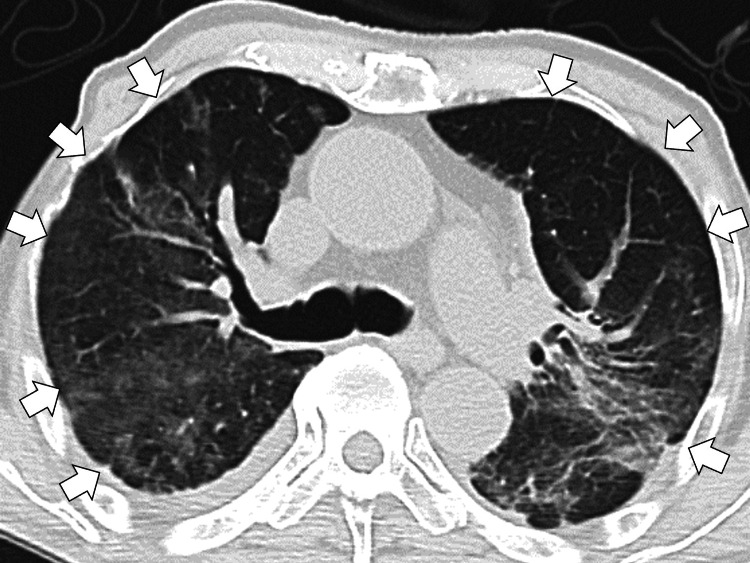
Follow-up chest computed tomography revealing near-complete resolution of ground-glass opacities (white arrows)
